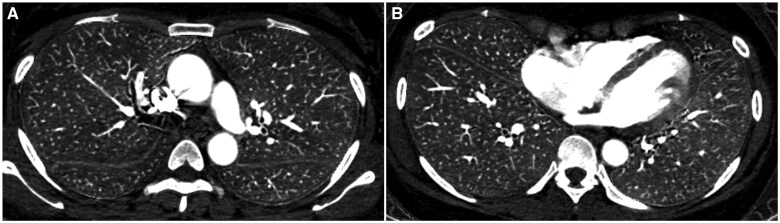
Iodine maps (upper zone [A] and lower zone [B]) of a 42-year-old female patient with a body weight of 64 kg. This patient underwent CT pulmonary angiography at the mid-inspiratory level. A contrast agent with an iodine concentration of 370 mgI/mL was injected from the right antecubital vein. The iodine concentration of the right upper, right middle, right lower, left upper and left lower lobes, and main pulmonary artery was 1.12, 1.09, 1.16, 1.20, 1.67, and 15.9 mgI/mL, respectively.
